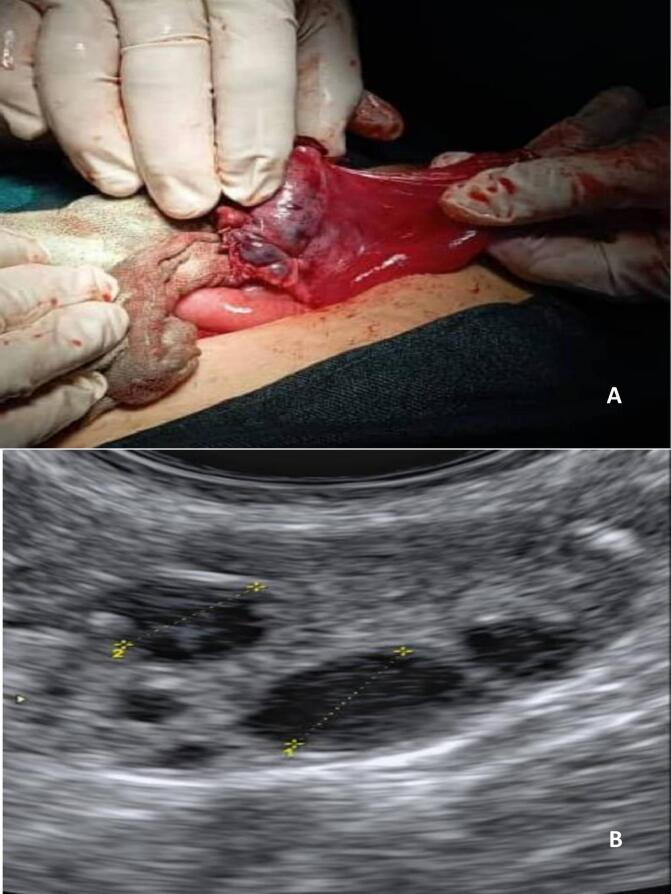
A: Ovarian preservation was achieved after confirming reperfusion and restoration of the ovary's normal reddish coloration. B: On day 13 of the subsequent menstrual cycle, a dominant follicle was identified on vaginal ultrasound diameter 3 cm.
